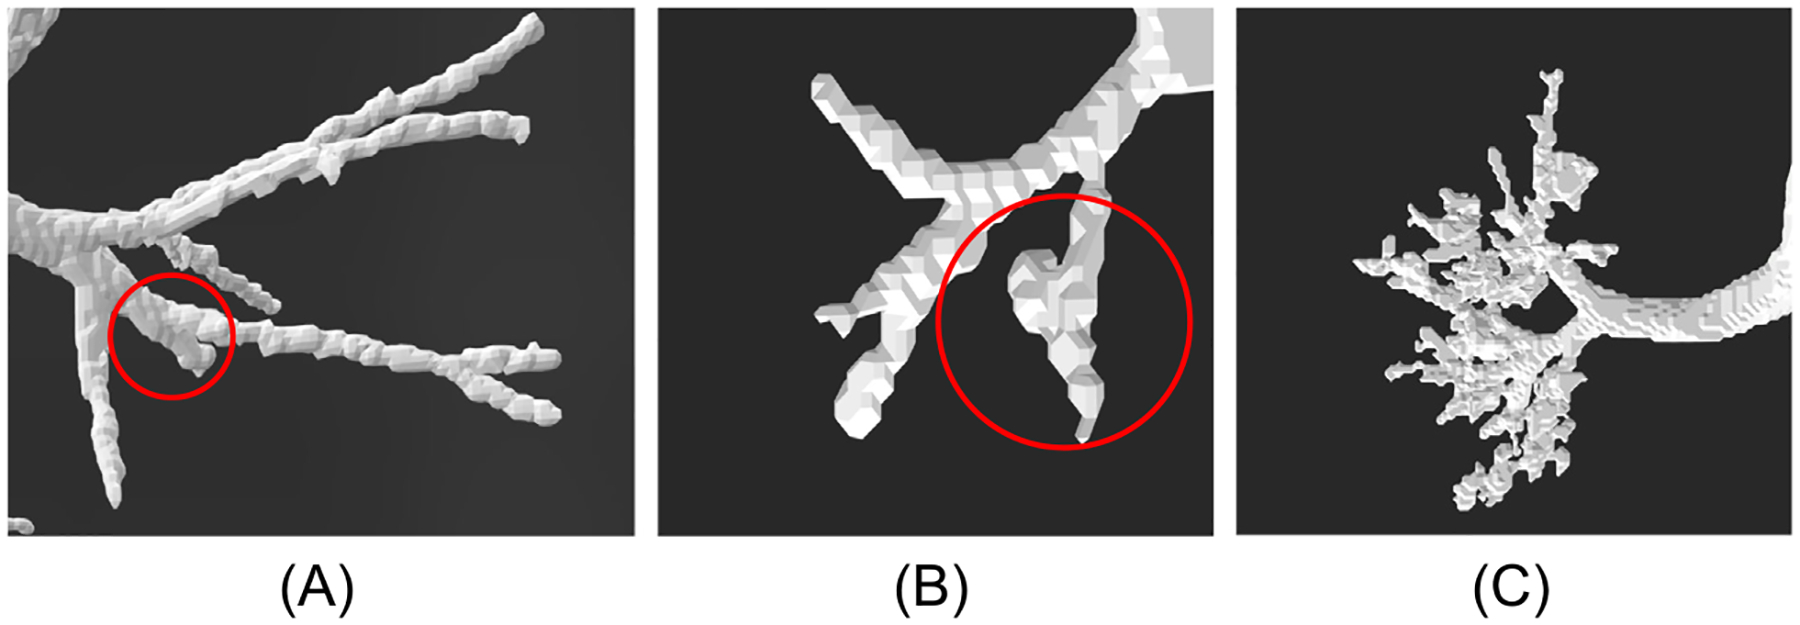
Examples of the criteria applied to discard geometries after manual visual inspection. (A) Example of a non-physical bronchi, where the algorithm incorrectly tried to continue the previous generation of bronchi in a direction that did not exist in the CT scan. (B) Example of a non-physical terminal bronchi. (C) Example of an unrealistic segmentation of the tracheobronchial tree, due to motion artifacts in the lungs.
